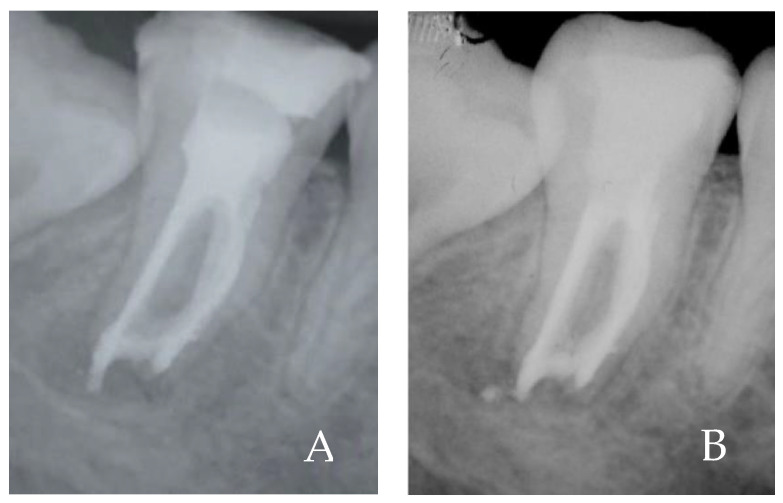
(A) Post-operative X-ray with root canal obturation with pre-plasticized gutta-percha. (B) 4-month follow-up X-ray showing good healing.
